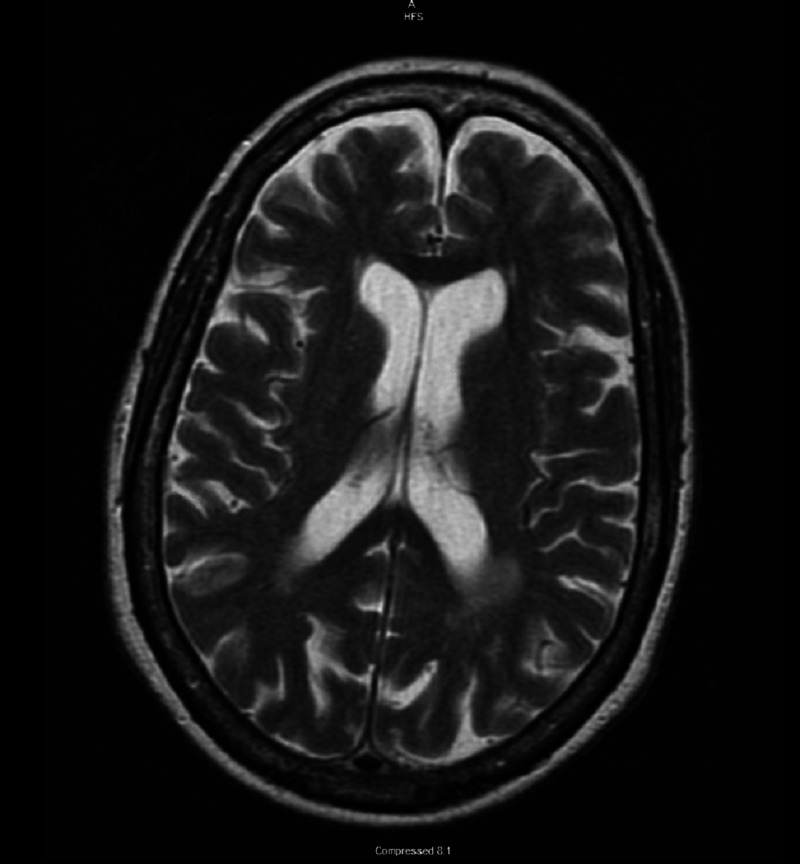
Magnetic resonance imaging (T2 hyperintensity) of brain showed non-specific white matter changes, no acute abnormalities.
